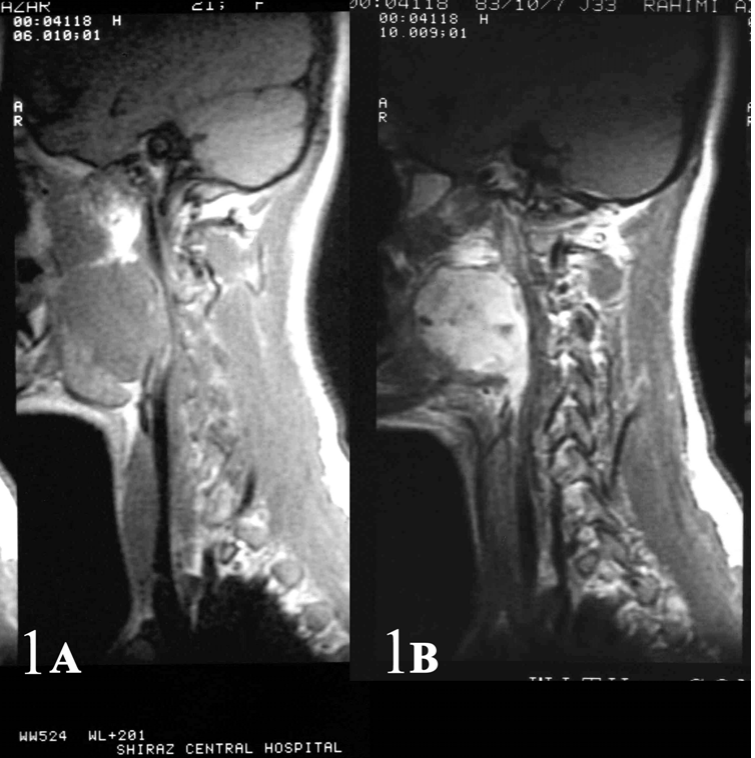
AB: Sagital and coronal (2AB) contrast-enhanced MR images of the right parapharyngeal synovial sarcoma.
